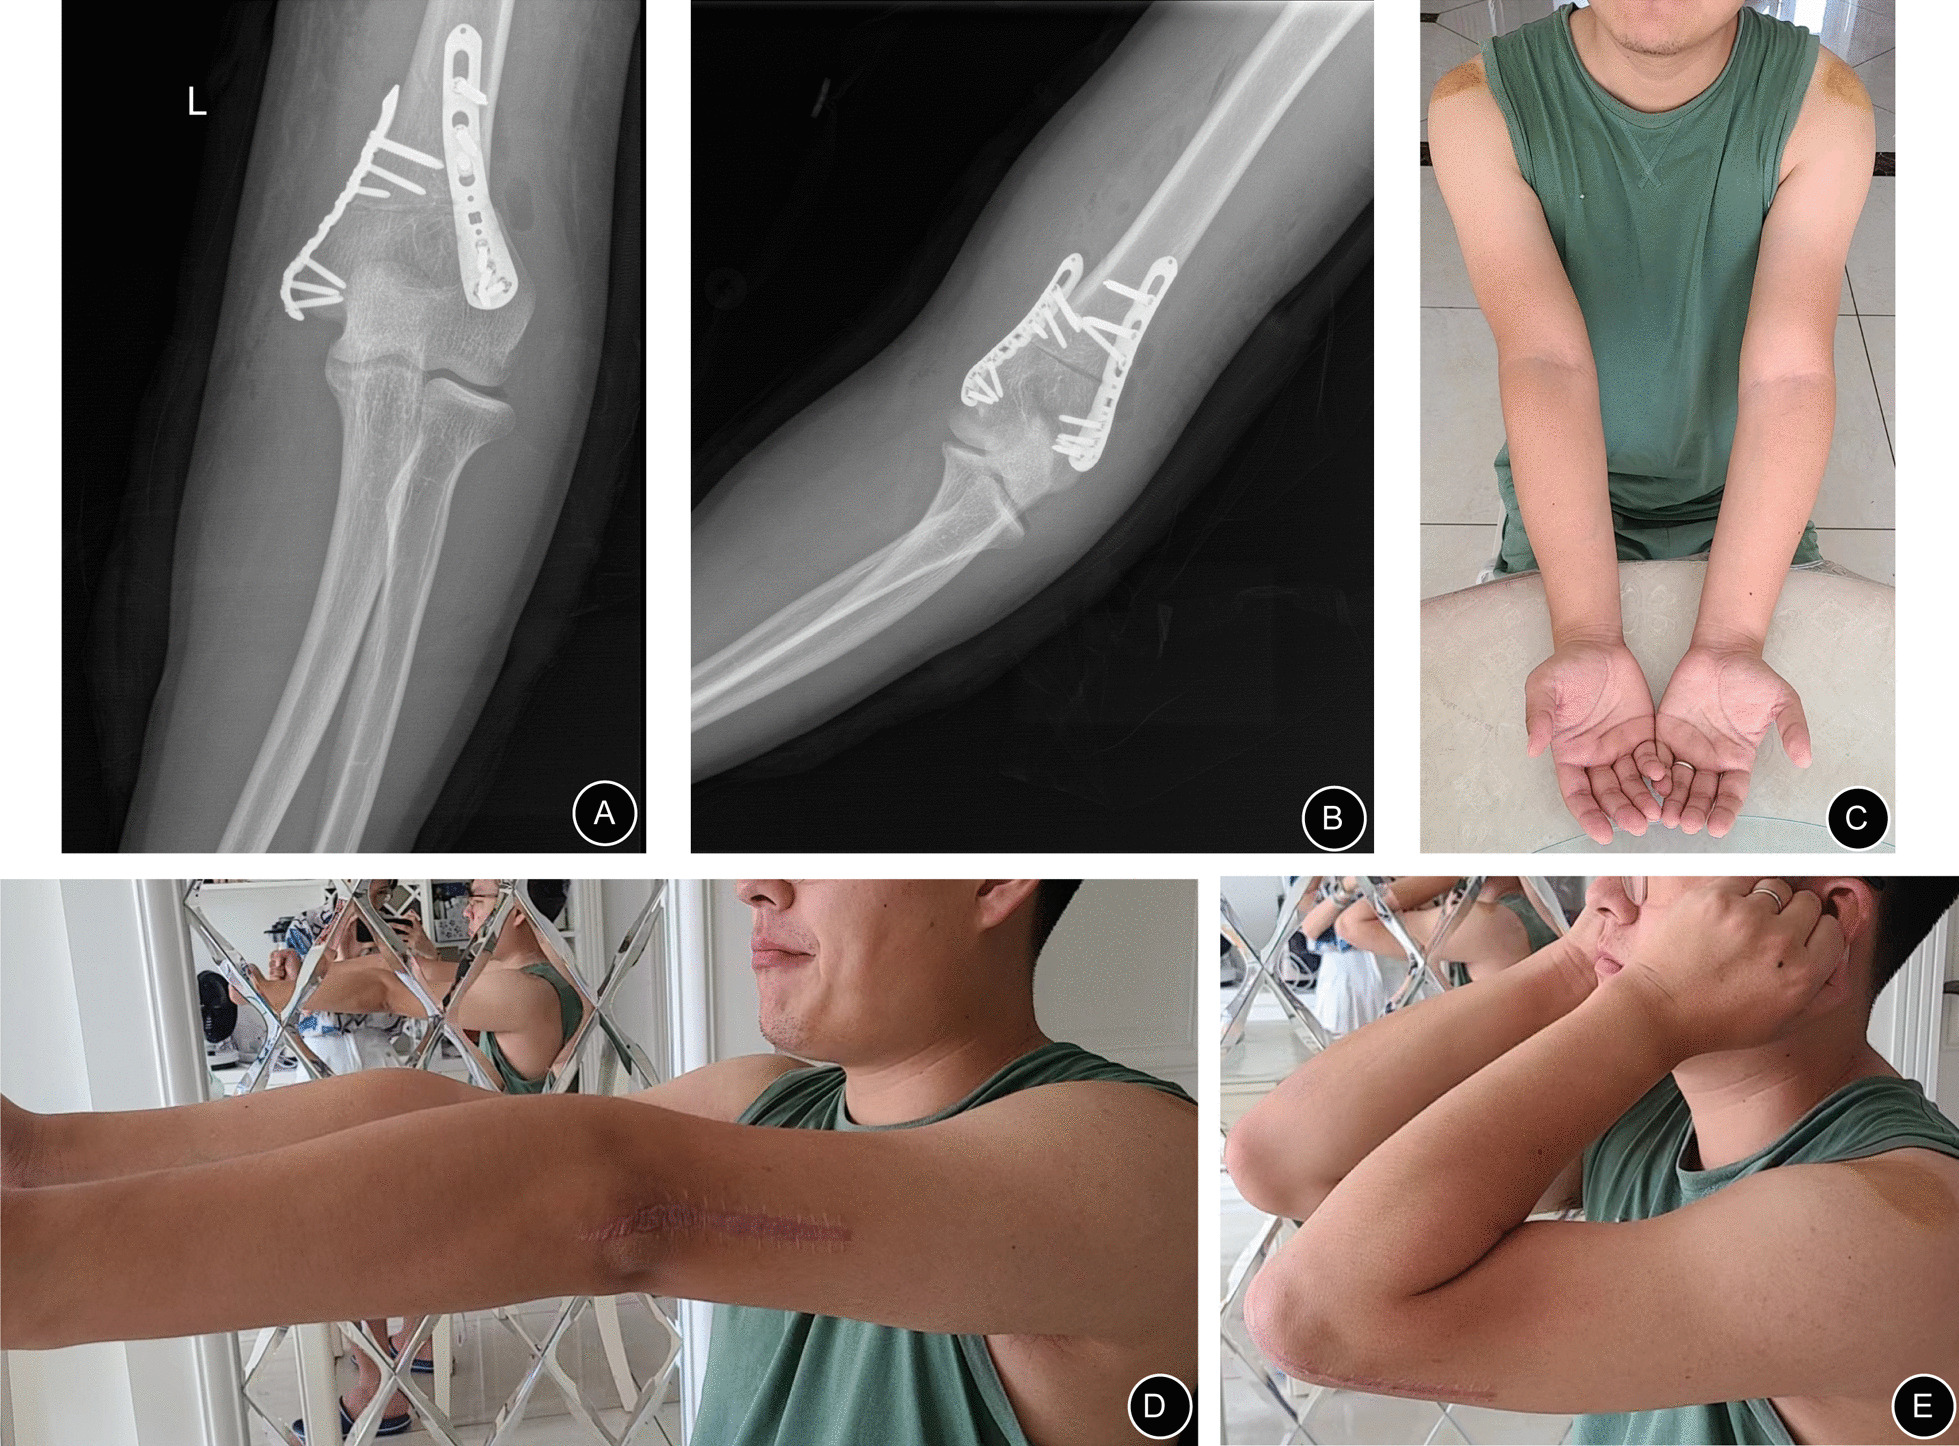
A 24-year-old man presented with a left cubitus varus deformity after surgery (Case 5): A, B Appropriate correction was achieved as evident on the postoperative anteroposterior and lateral radiographs. C The varus deformity was corrected. D, E The range of flexion and extension of the bilateral elbow joints after surgery remained unchanged (0°–150°)
